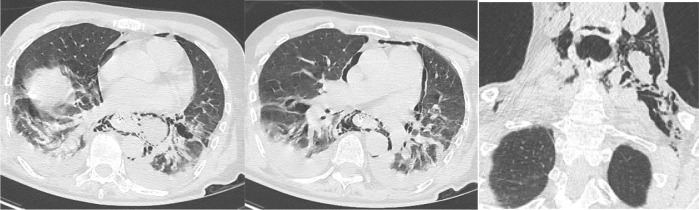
Chest CT. Axial views in the lung window and coronal reformatting demonstrating cervical subcutaneous emphysema and pneumomediastinum. Source: Charleroi University Hospital.
